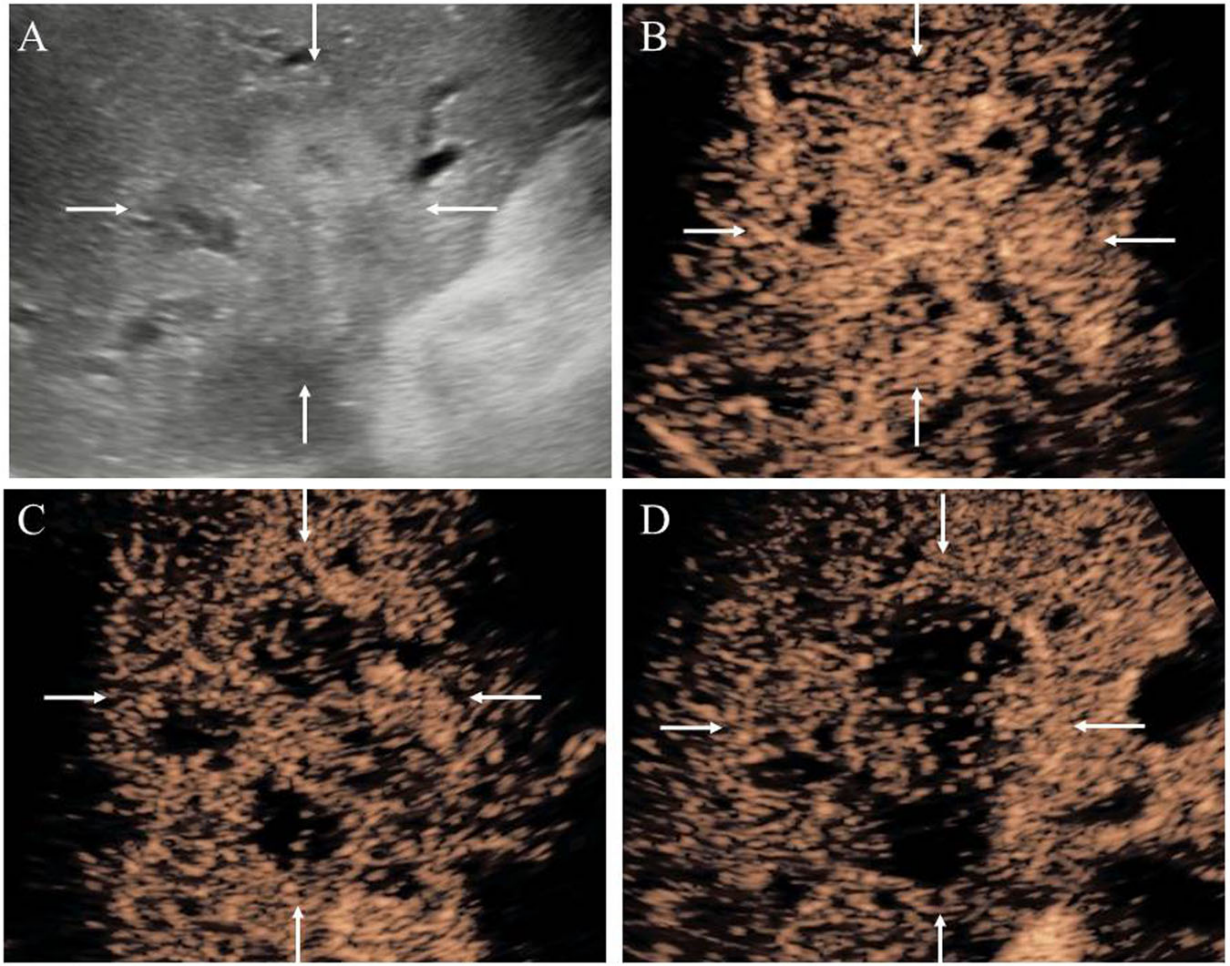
A 50-year-old woman with a 35 mm intrahepatic cholangiocarcinoma (ICC) lesion which is confirmed by pathology as LD-type. A B-mode ultrasound shows a hyper-echoic lesion (white arrow) with ill-defined boundary located in the perihilar area. B Arterial phase hyperenhancement (APHE, white arrow) is observed at 17 s after contrast agent injection. C Early wash-out is observed at 29 s (white arrow). D Marked wash-out is present at 85 s (white arrow)
